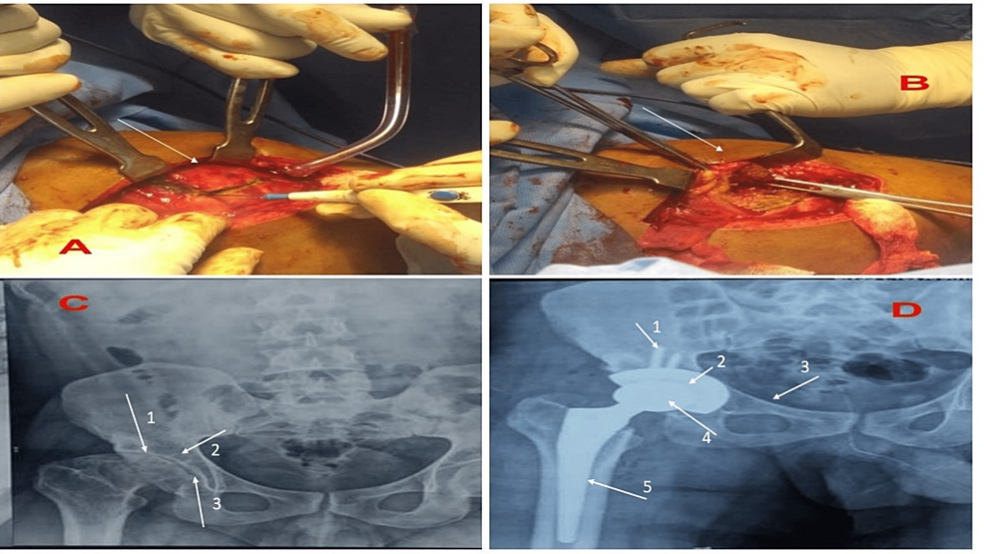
The lateral gluteus medius-sparing approach A: the arrow shows the incised anterolateral fibers of the vastus lateralis with fibers of the gluteus medius. B: the arrow shows the raised gluteus medius flap. C: radiographic images; arrow 1 shows subchondral cyst and sclerosis; arrow 2 shows the reduced joint space with a deformed femoral head; arrow 3 shows osteophytes in the femoral head. D: radiographic images; arrow 1 shows the acetabular screw; arrow 2 shows the acetabular metal shell; arrow 3 shows superior pubic rami; arrow 4 shows the prosthetic femoral head; and arrow 5 shows the femoral stem
